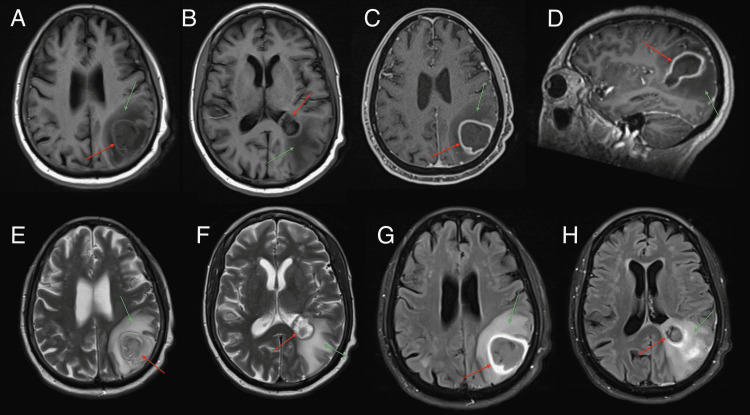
Magnetic resonance imaging of the brain with and without intravenous contrast. The images show a 3.2 X 3.2 X 3.1cm rim-enhancing left parietal lesion likely to reflect an abscess (red arrows) with vasogenic edema and mass effect (green arrows). (A and B) Both axial views are T1-weighted without contrast; (C) axial view and (D) sagittal view are T1-weighted with contrast highlighting the rim-enhancing lesion. (E and F) both axial views are T2-weighted images. (G and H) both axial views are FLAIR images. FLAIR: fluid-attenuated inversion recovery
